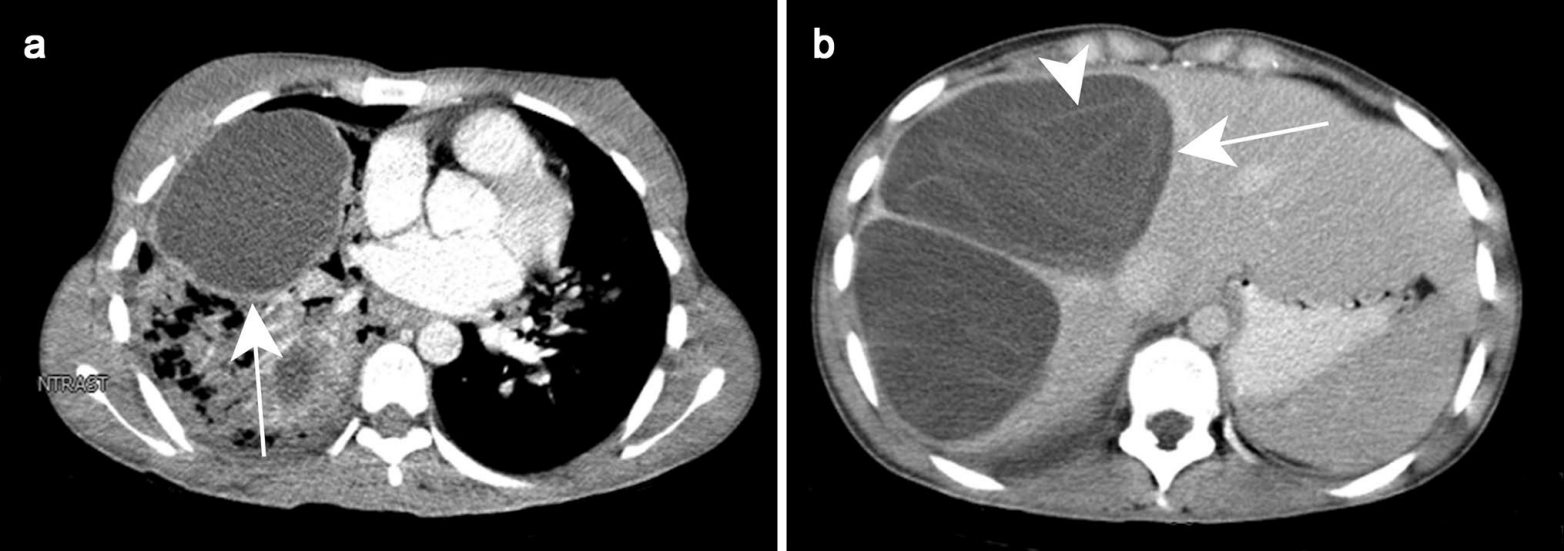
Cystic echinococcosis type CE3a in a 27-year-old female patient with cough. Axial contrast-enhanced CT of the lower pulmonary parenchyma a reveals a pulmonary cyst in the middle lobe (white arrow) associated with a partial consolidation of the right lower lobe. Axial contrast-enhanced CT of the upper abdomen b shows two hepatic cystic lesions (white arrow) with internal detached membranes (“water-lily” sign) (white arrowhead)
